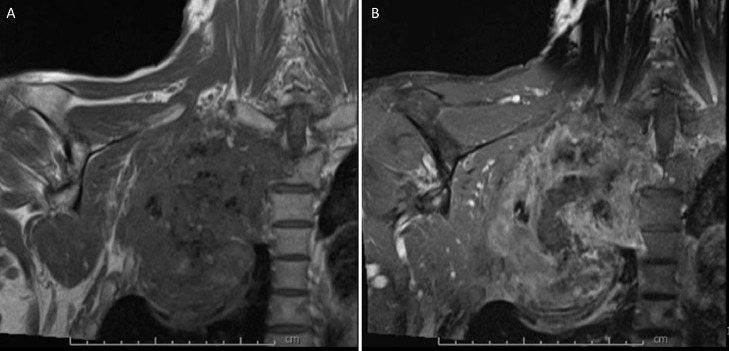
MRI brachial plexus protocol, T1 coronal (A), and T1 coronal post gadolinium (B) demonstrates a T1 isointense peripherally enhancing right upper chest wall mass extending into the right axilla, and eroding right second rib
